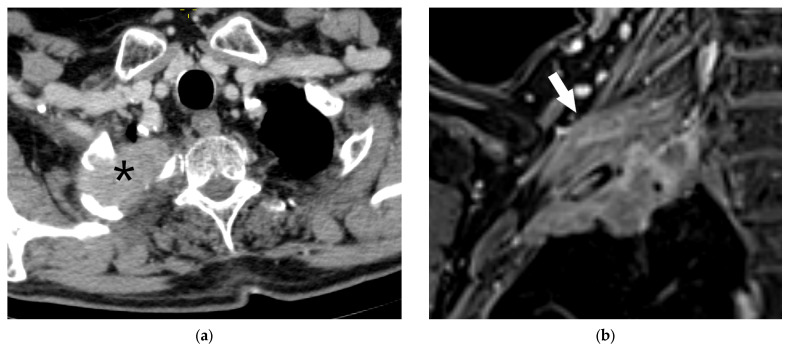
Pancoast tumor: Axial contrast-enhanced chest CT image shows a Pancoast tumor (asterisk) at the upper-right lobe’s apex (a), with chest wall invasion and rib erosion. A coronal post-contrast fat-saturated T1-weighted MRI image (b) confirms the invasion of the brachial plexus at the C7 to T1 level (arrow, T4 category).
